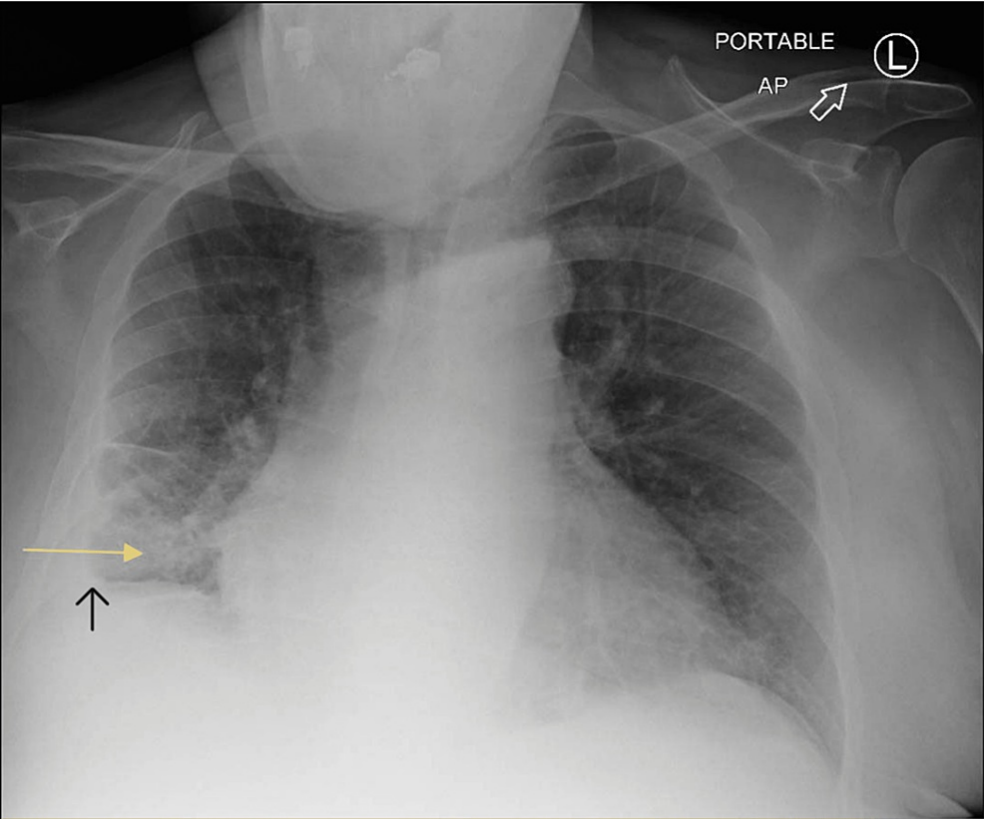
Chest X-ray The yellow arrow shows right base airspace disease and the black arrow shows right-side pleural effusion
